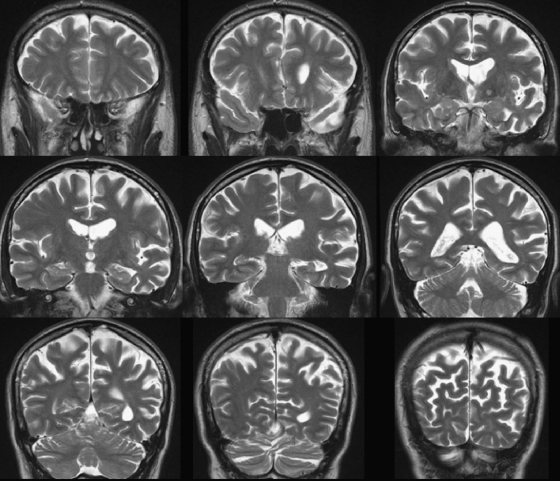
Coronal FLAIR magnetic resonance sections of the patient's brain (left hemisphere shown on the right) 3 years after symptom onset, showing predominant left fronto-temporo-parietal atrophy.
